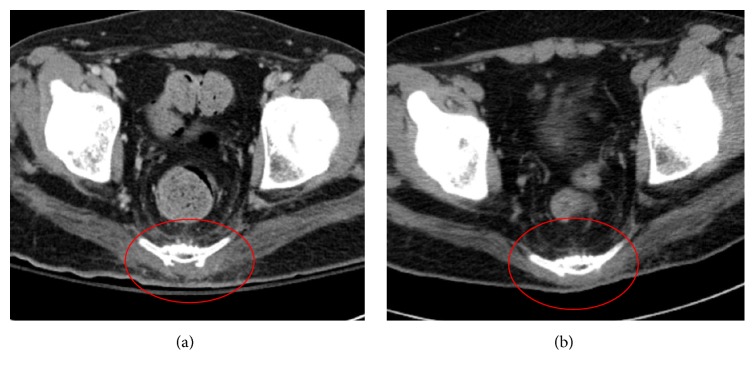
(a) Computed tomographic (CT) image at 3 weeks postoperatively. (b) CT image at 5 months postoperatively, showing maintenance of muscle depth with no change.
